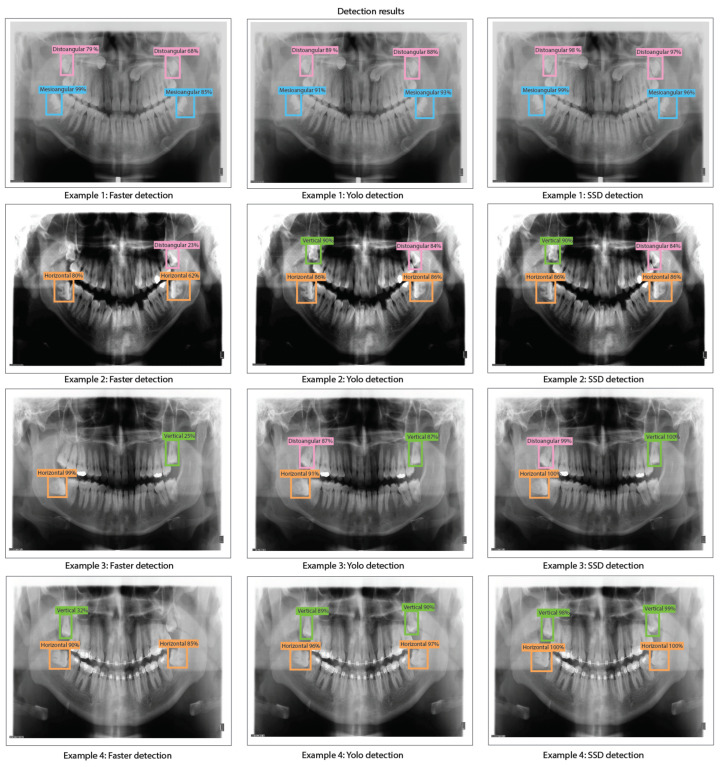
Third molar angle detection in dental panoramic X-rays with ResNet-18 as feature extractor for Faster R-CNN, YOLO v2, and SSD.
